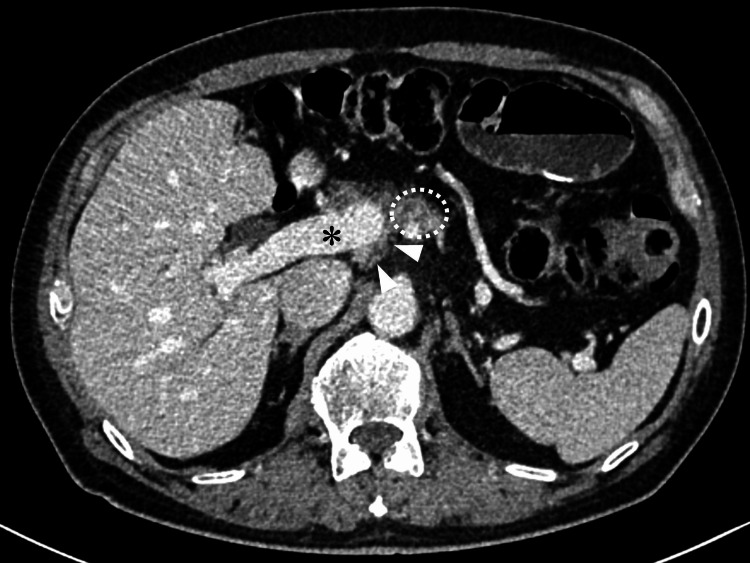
The contrast-enhanced computed tomography image before neoadjuvant chemotherapy Arrowheads indicate the dorsal pancreatic parenchyma; the asterisk indicates the portal vein; dotted lines outline the pancreatic tumor.
